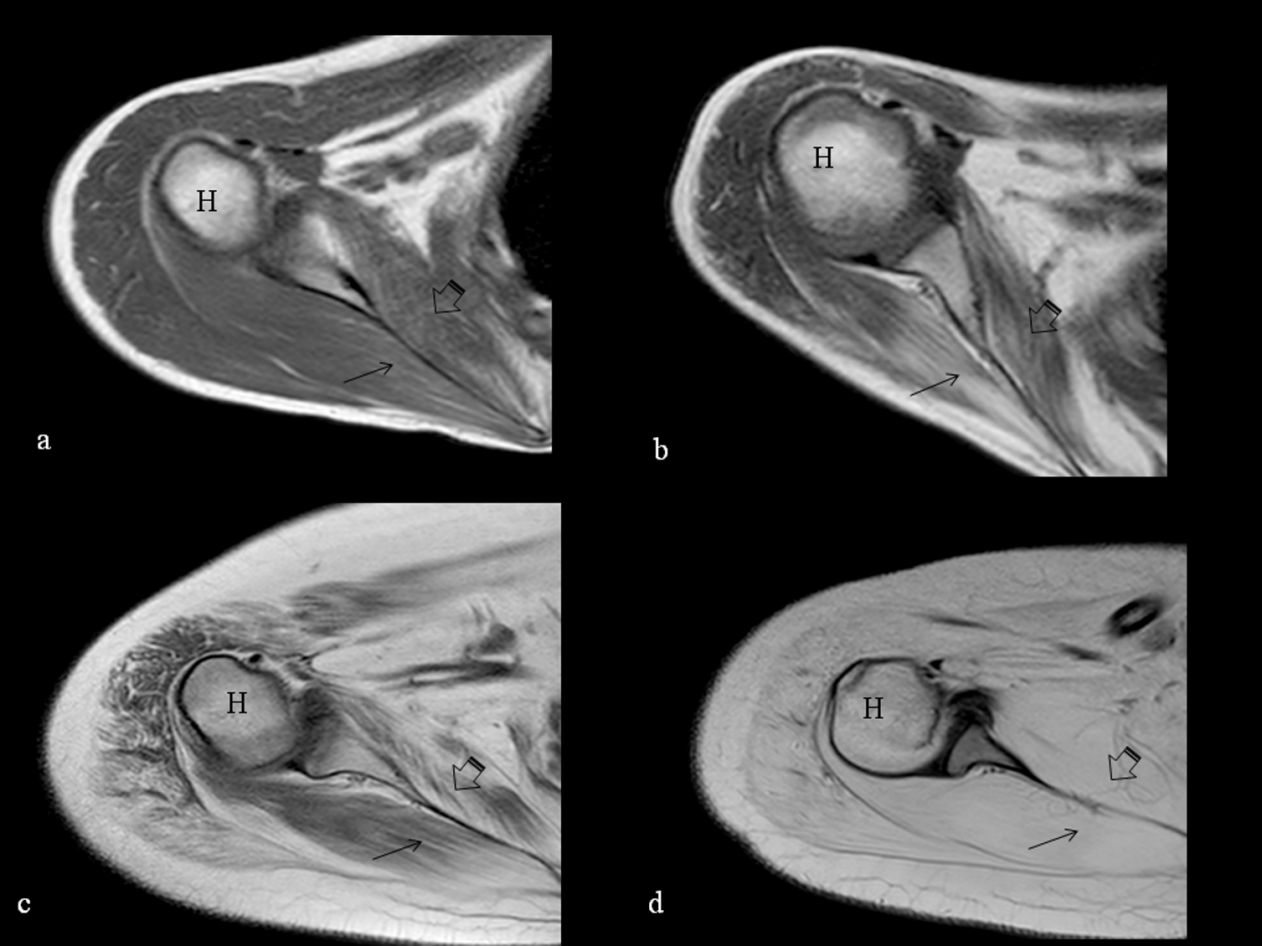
T1-weighted axial MRI of the shoulder. T1-weighted axial MRI of the shoulder at the level of the humeral head (H) showing increased signal suggestive of fatty replacement in the muscles at this level. The involvement is milder in the youngest ambulant patients aged 10 and 11 (a and b respectively) with more evident fatty replacement in the subscapularis (arrowhead) and infraspinatus (arrow) and more marked in the older non-ambulant ones, both 17 years old (c and d), with severe involvement of all the muscles shown in image d.
